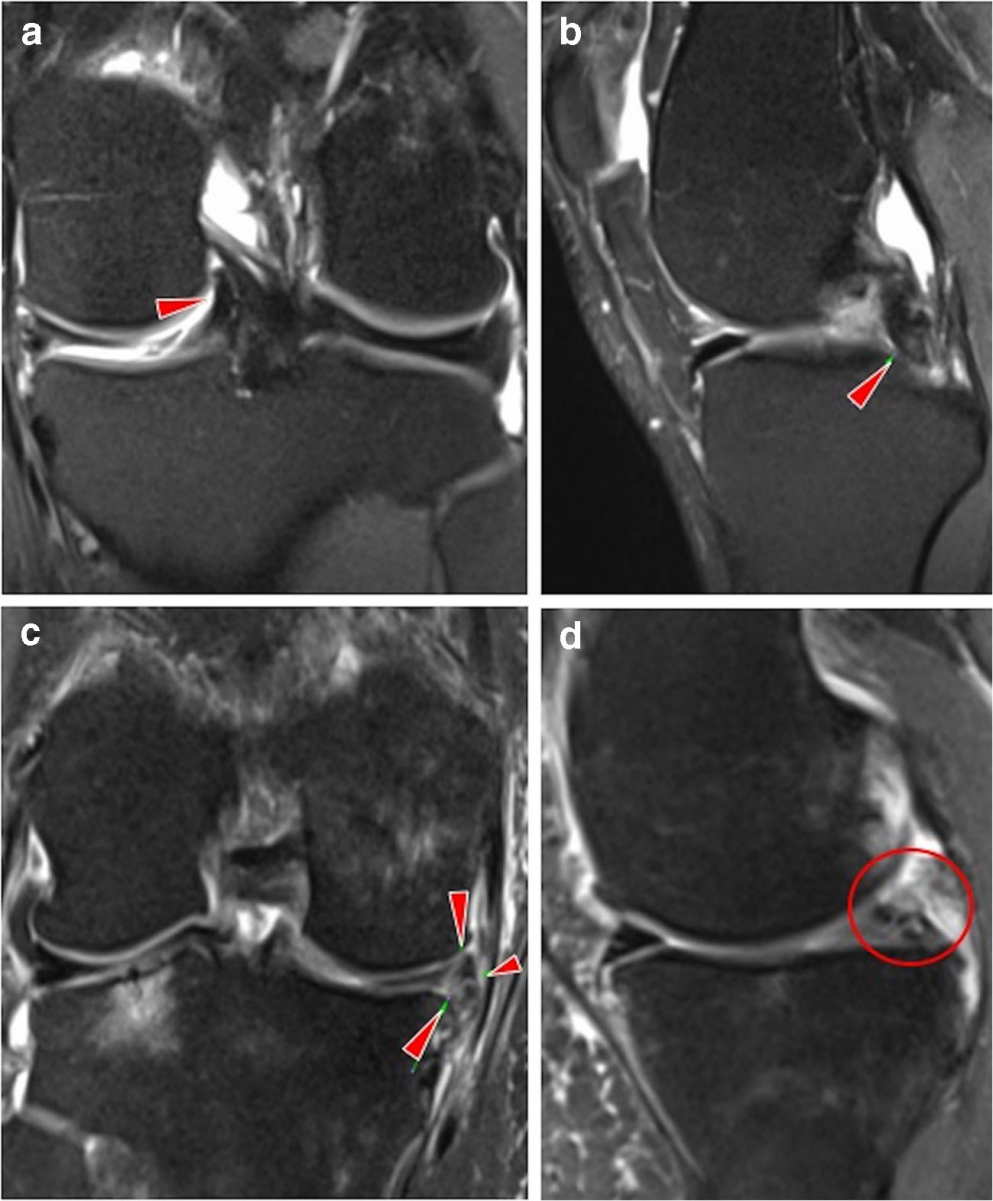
Coronal proton-density fat-saturated MR images (a, c) and sagittal images (b, d) demonstrate bucket handle tear (a, b; arrowheads) in the left knee of a 54-year-old man, and complex macerated (c, arrowheads; d, circle) meniscal tear in the right knee of a 57-year-old woman
